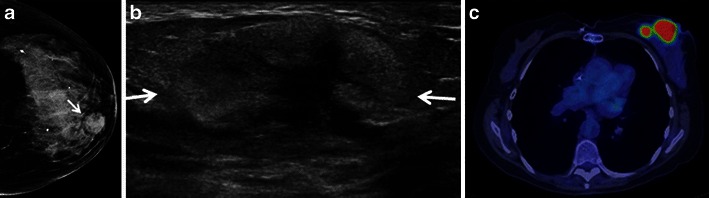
A 74-year-old woman with primary large B cell breast lymphoma presenting with a palpable mass in the left breast. a Cranio-caudal left breast mammogram shows an isodense circumscribed mass (*arrow*) in the medial left breast. b Ultrasound of the palpable mass demonstrates an oval, circumscribed principally echogenic mass with an irregular shaped central area of hypoechogenicity (*arrows*). c PET/CT shows two areas of homogeneous hypermetabolic uptake in the medial left breast. No axillary lymph node uptake was seen (*not shown*)
